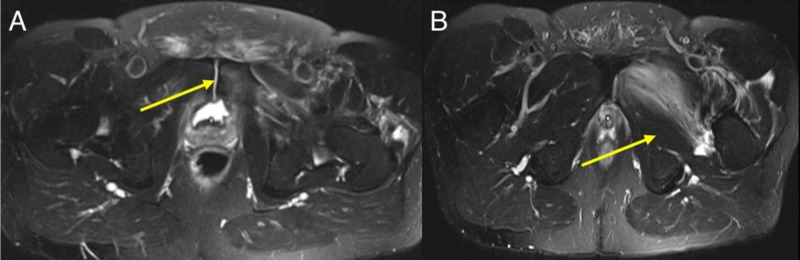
(A) MRI image shows fluid signal which appears to communicate from the bladder neck via the pubic symphysis inferolaterally into the left adductor collection with an adductor aponeurosis cleft sign noted. (B) Another MRI image shows a left-sided intramuscular collection with a maximally 6.5-cm transverse dimension and a 2.3-cm depth fluid collection noted centred upon the left adductor magnus muscle in the proximal thigh with marked surrounding oedema also involving the adductor brevis muscle.
